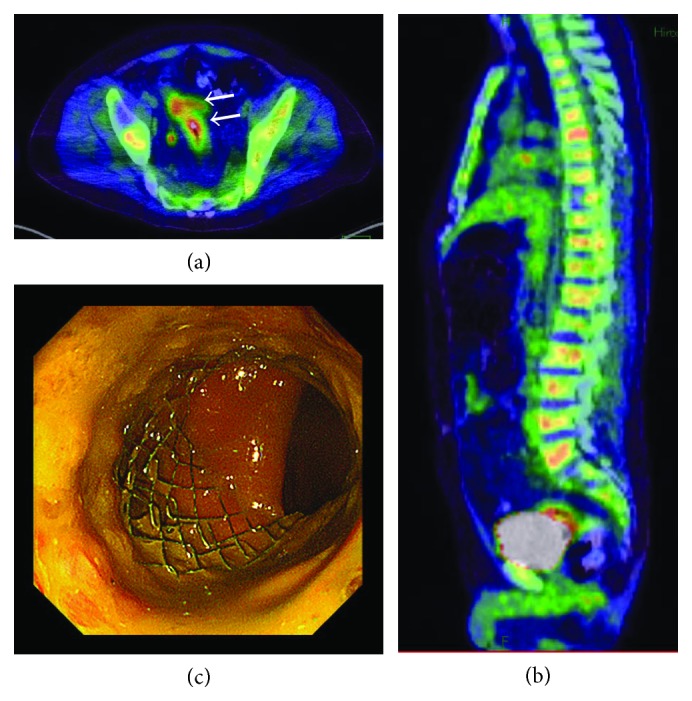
(a and b) CT scanning with positron-emission tomography shows significant FDG uptake in the rectum (arrows) and extensive bone metastasis in the pelvis and vertebrae. (c) The self-expandable metal stent was placed endoscopically.
